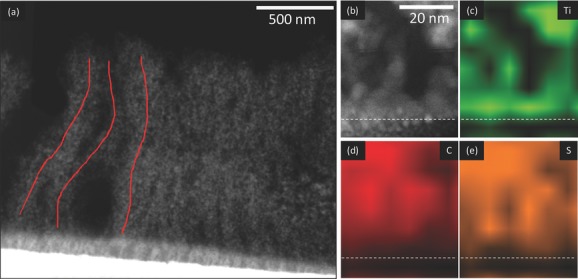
a) Cross-sectional STEM HAADF view of the photoanode, with some of the trees highlighted in red. b–e) Dark field STEM and X-ray elemental maps (respectively Ti, C and S) from the base of the TiO_2_ structures, showing presence of sulphur and indicating a good infiltration of P3HT inside the titania network. The white dashed line outlines the surface of the substrate.
